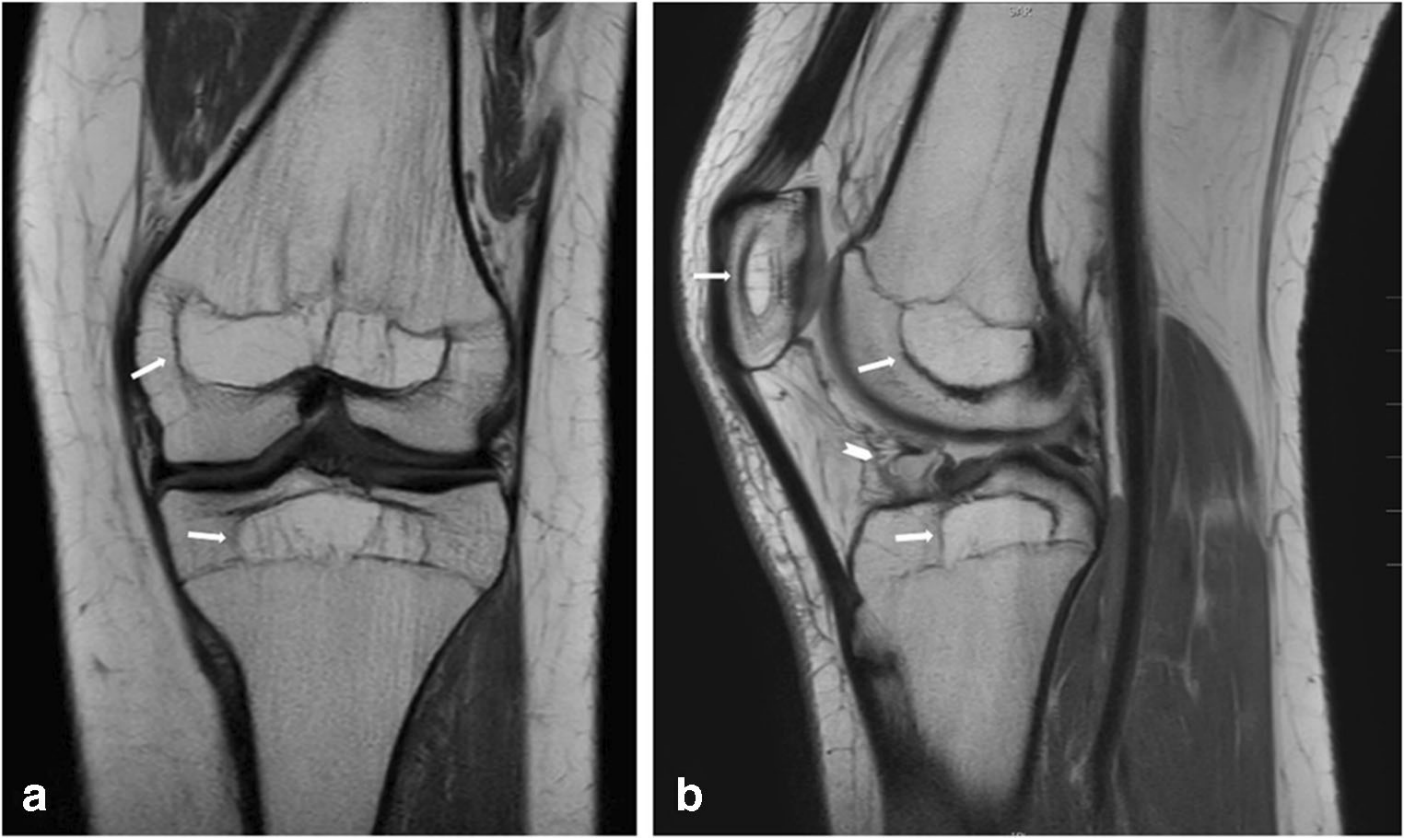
a–b MRI of the left knee. a Coronal T1-weighted and b sagittal PD-weighted images. The former SOCs are clearly delineated by low signal intensity lines in the epiphyses of the distal femur, proximal tibia, and patella; all SOCs display higher internal signal intensity than surrounding bone marrow with sparse trabeculation. Notice the absence of low signal intensity growth recovery/arrest lines in the metaphyses
